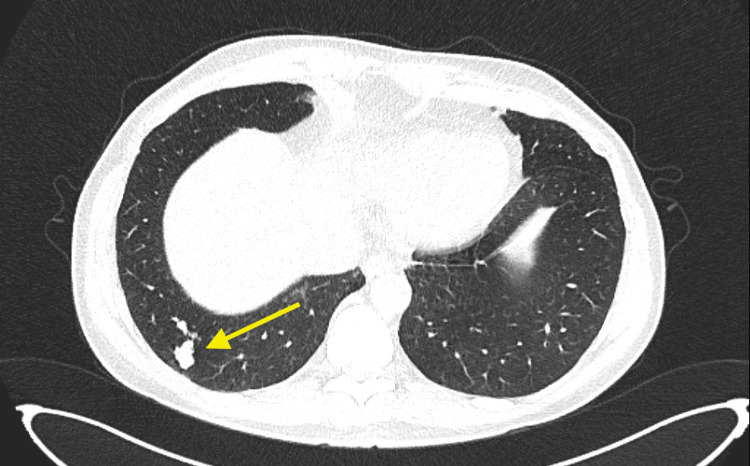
Chest CT with multiple nodules Chest CT with multiple nodules 0.8-2.0 cm. Arrow points to a solid nodule in the right lung.
